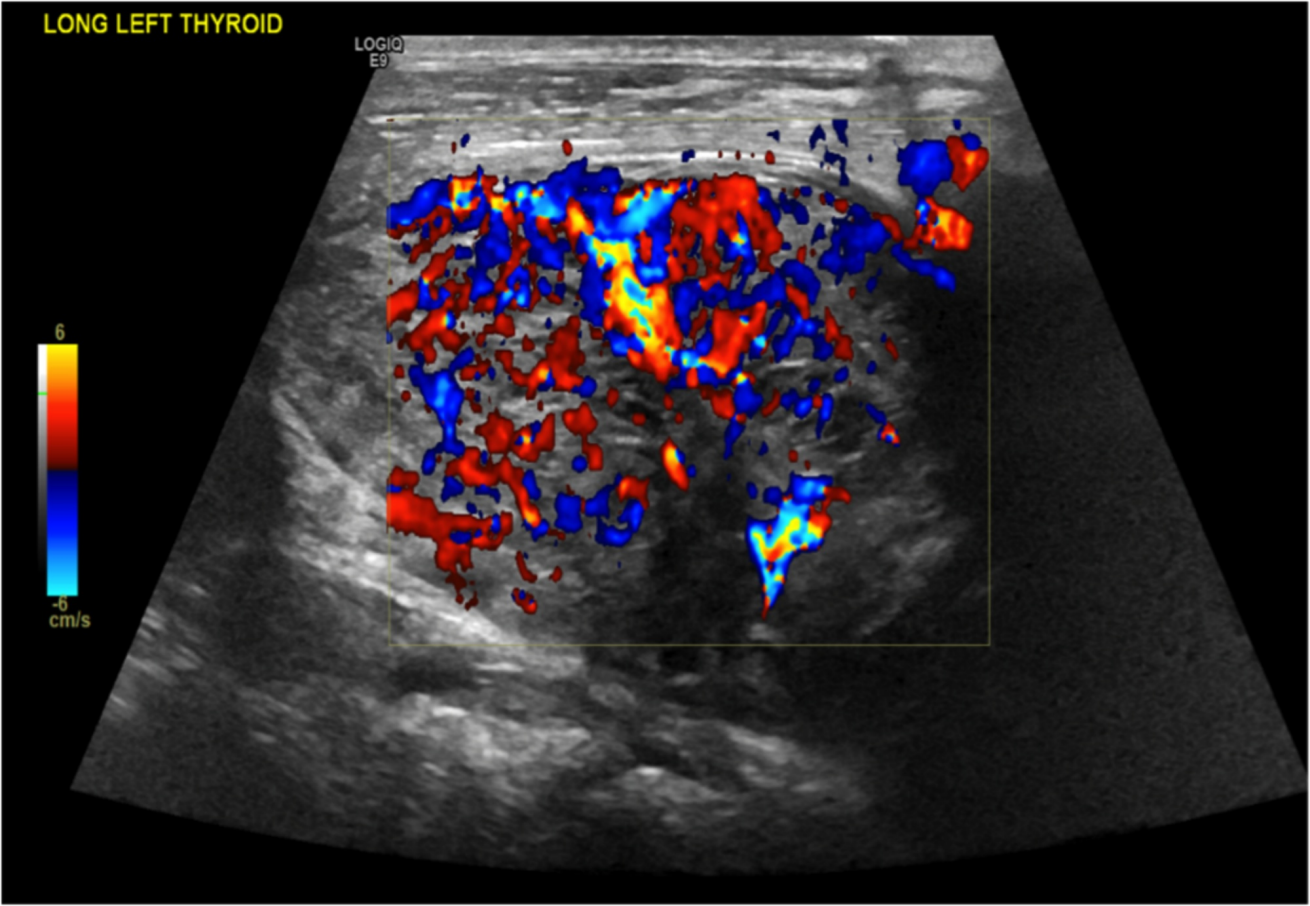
Doppler showing vascularity in the left thyroid lobe
